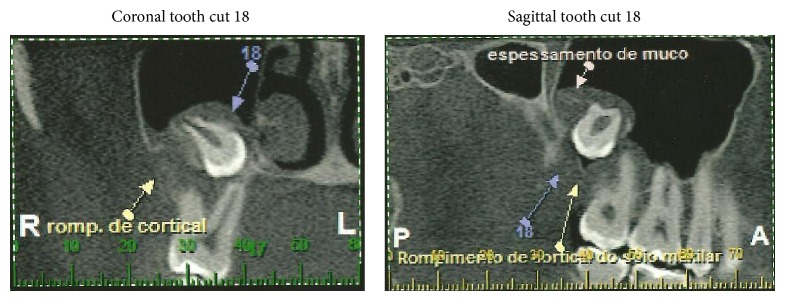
Computed tomography examination of the maxilla by volumetric acquisition to better locate DU 18.
